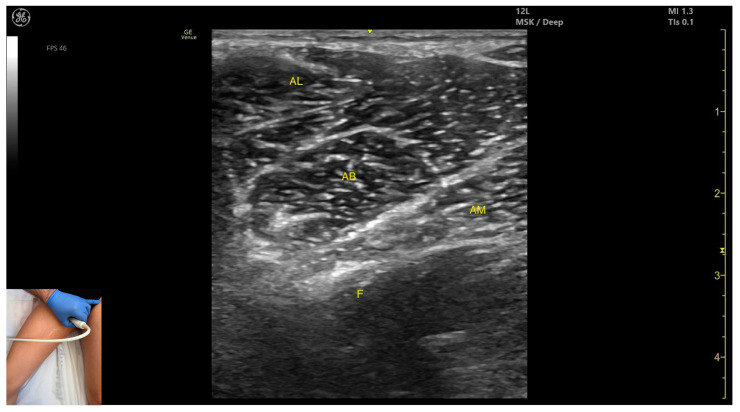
Ultrasound anatomy of *adductor longus* (AL) with key landmarks: AL—*adductor longus*; AB—*adductor brevis*; AM—*adductor magnus*; F—femur.
